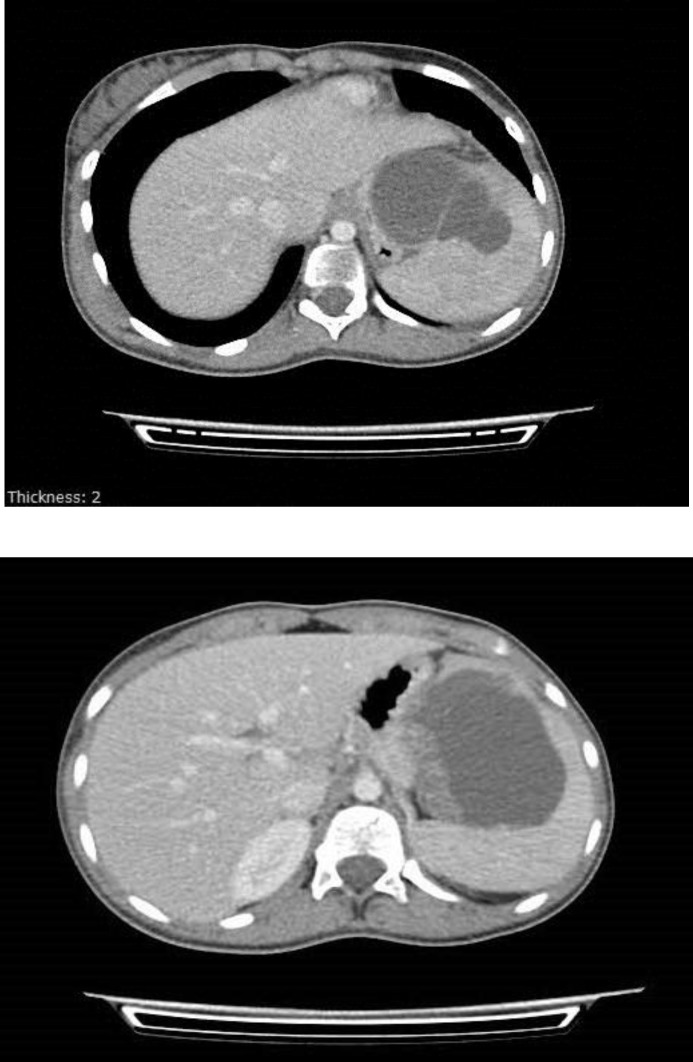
CT of the abdomen shows a splenic cyst
